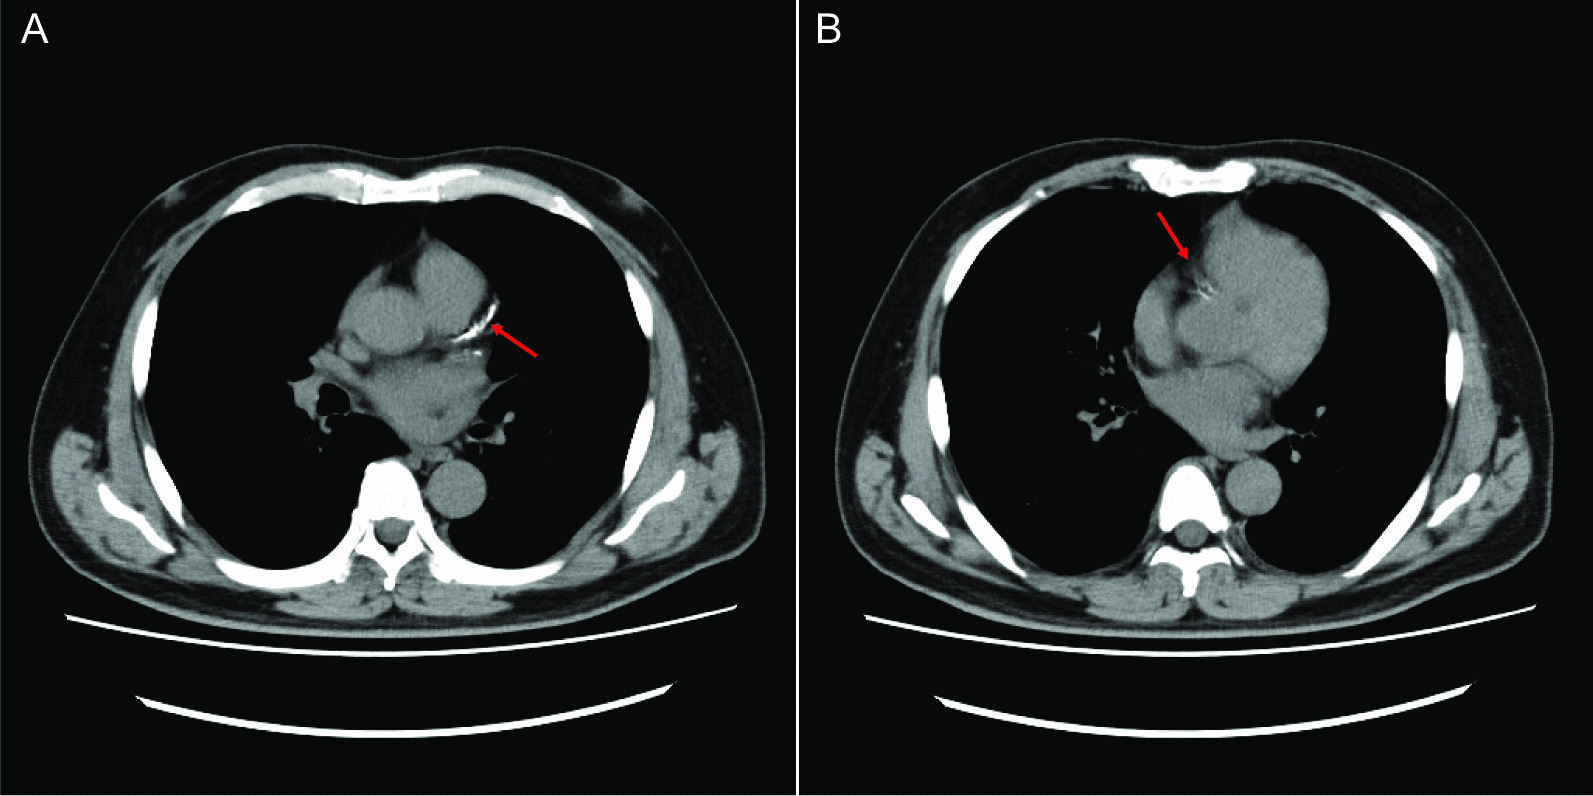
Computed tomography 5 months prior to myocardial infarction. A Calcification of the left anterior descending artery is seen (arrow). B Slight calcification of the right coronary artery is seen (arrow)
